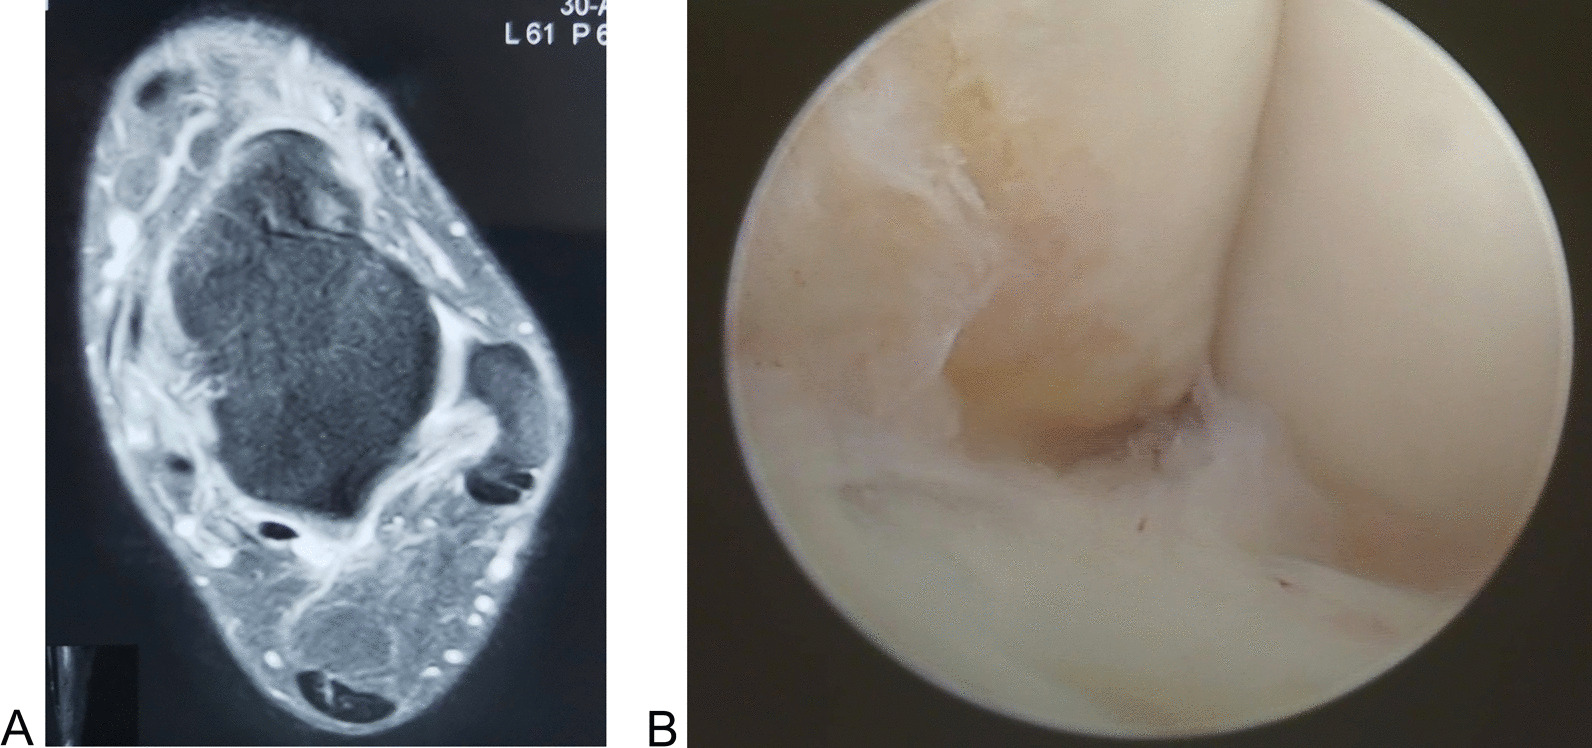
ATFL injury confirmed at the magnetic resonance imaging (A) and arthroscopy (B)
